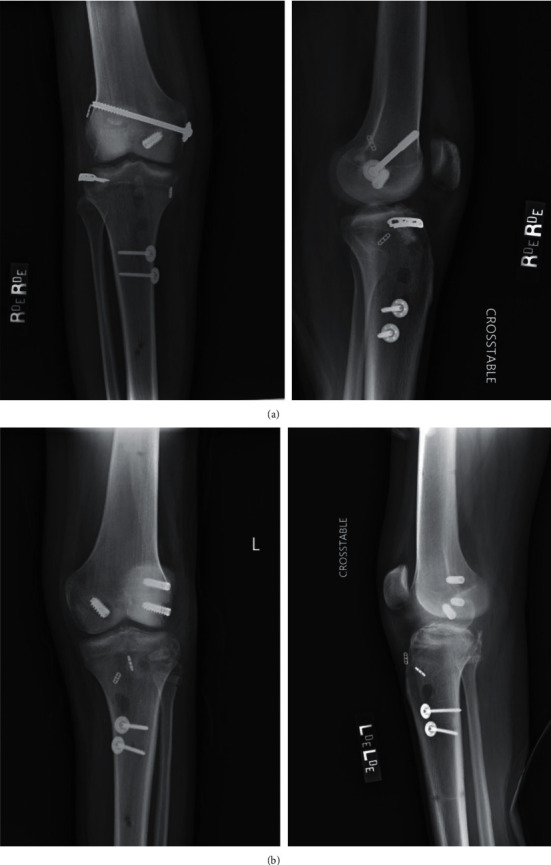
(a) Postoperative X-ray of the right knee. (b) Postoperative X-ray of the left knee.
